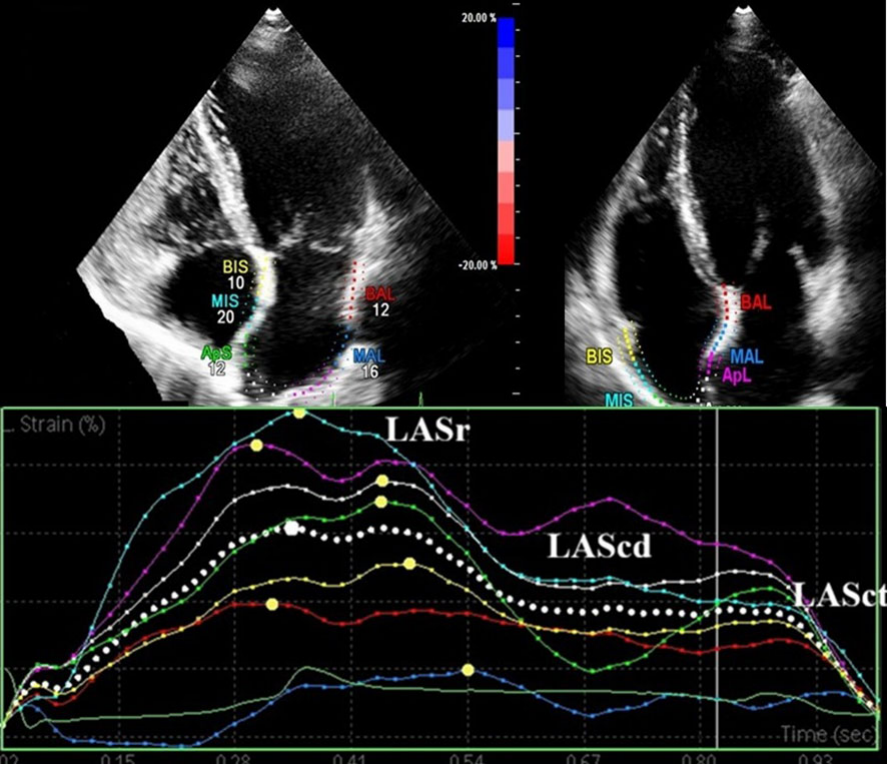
Apical four-chamber views of the left and right atria (top) and speckle-tracking–derived atrial strain curves (bottom) in a patient with atrial fibrillation.
